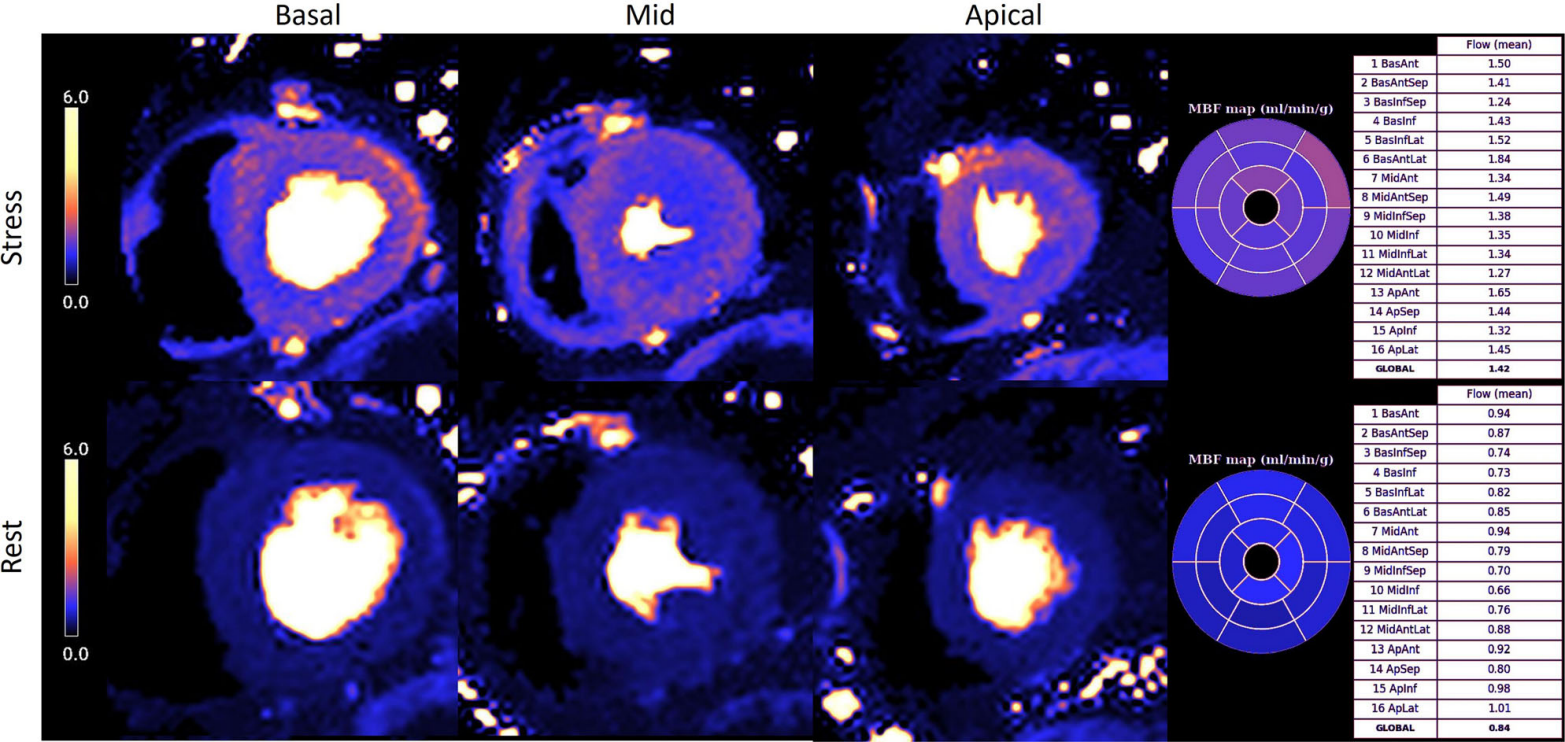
Example of abnormal perfusion scanning results on cardiac MRI. The stress perfusion images in the top row demonstrate a circumferential subendocardial perfusion defect when compared with those at rest in the lower row. The calculated global stress myocardial blood flow (MBF) is low at 1.42ml/g/min (abnormal < 2.25). Myocardial perfusion reserve (MPR) is also reduced at 1.69 (abnormal < 2.2). These results are indicative of CMD
